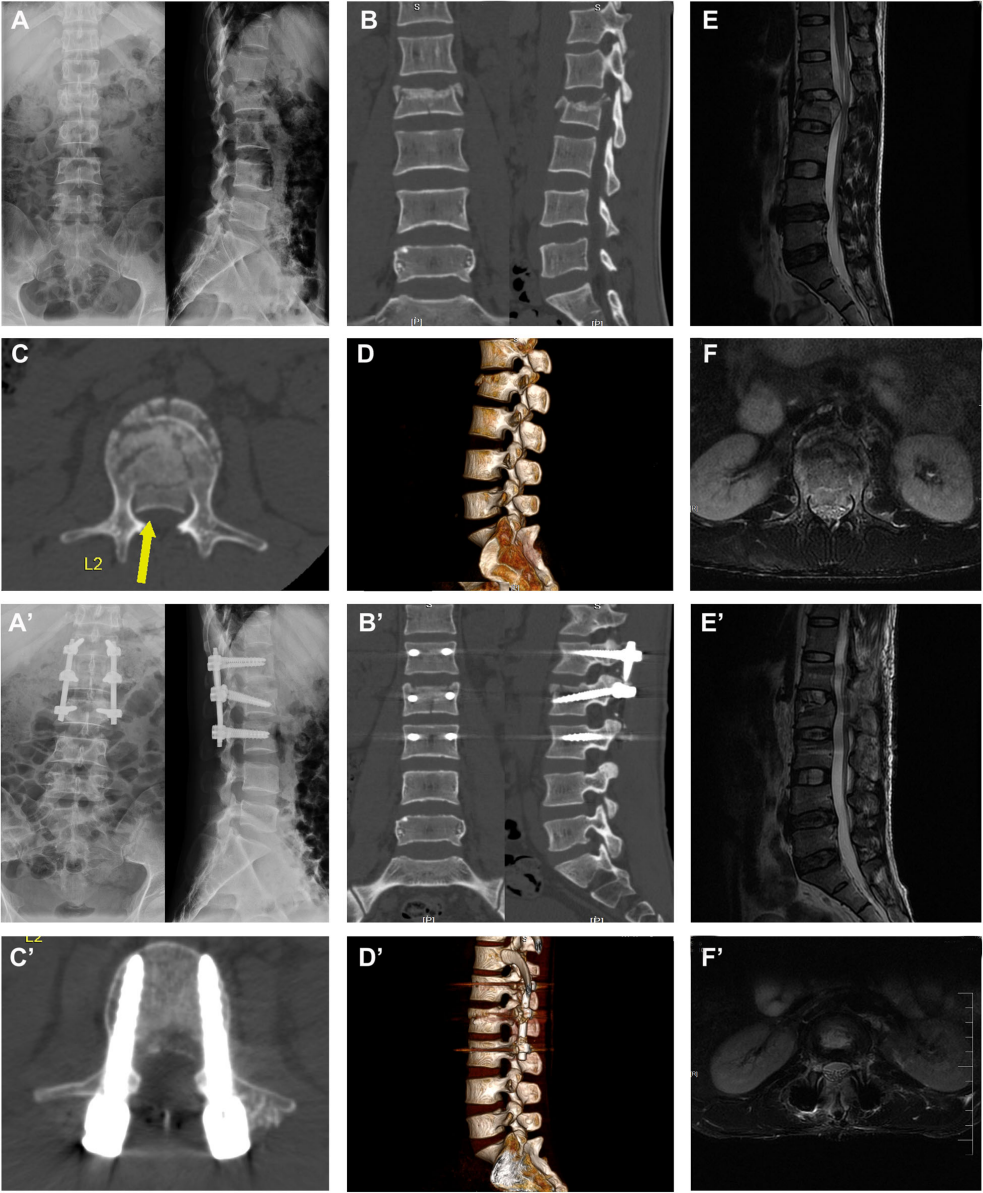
X-ray, CT, and MRI of a case with Denis type B TL fracture treated by SSIF-IA system. A, A’ Anteroposterior and lateral X-ray demonstrating the L2 compression fracture before and after operation. B, B’ Lateral spiral CT showing the L2 compression fracture with injured superior endplate before and after operation. C, C’ Axial spiral CT showing the spinal canal encroachment by fragments of the fractured vertebra before and after operation. D, D’ The 3D reconstruction of lumbar spine with the L2 compression fracture before and after operation. E, E’ Sagittal MRI confirming the L2 compression fracture with vertebra edema before and after operation. F, F’ Axial MRI confirming the spinal canal encroachment by fragments of the fracture vertebra along with posterior elements of the vertebra before and after operation. CT, computed tomography; MRI, magnetic resonance imaging; 3D, 3-dimensional
